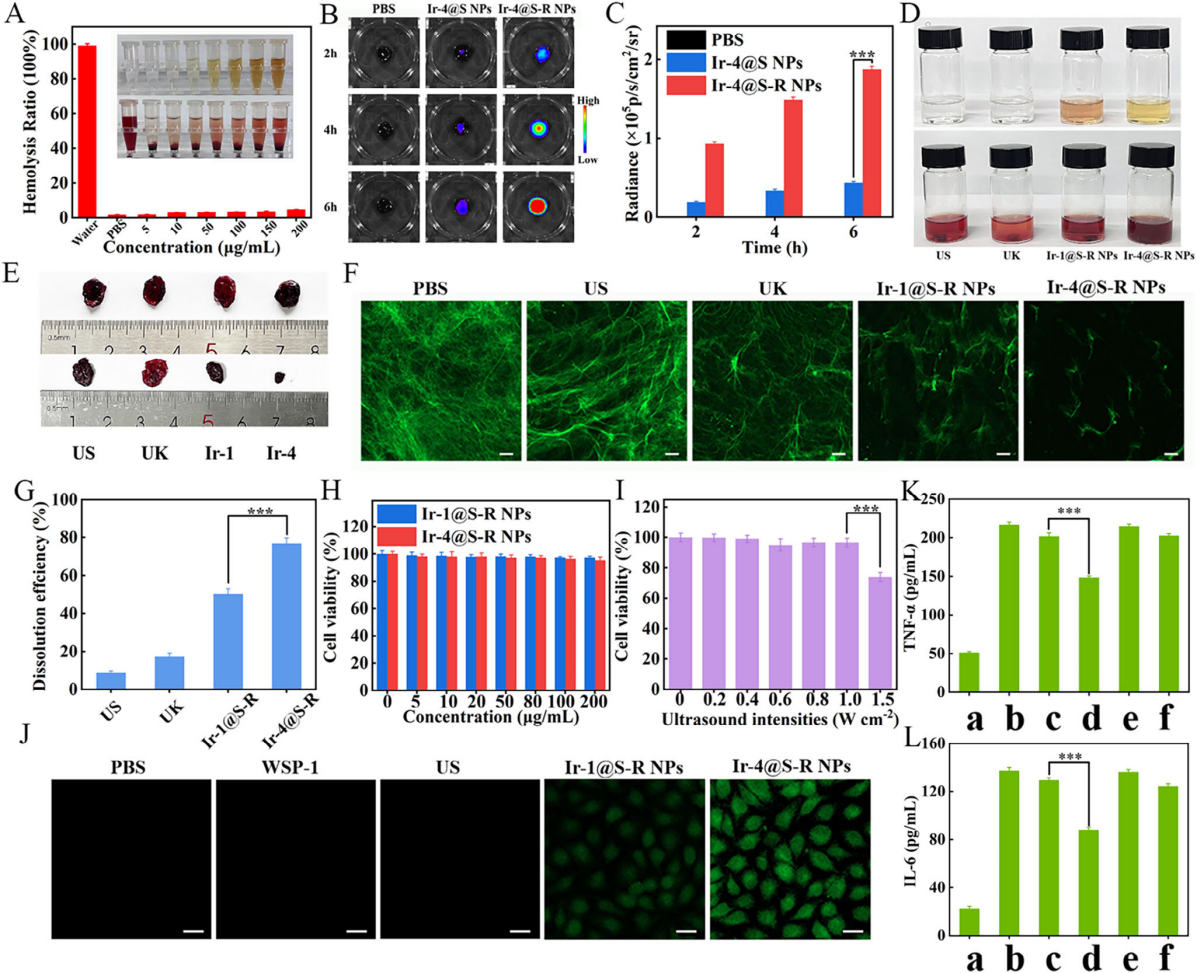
A) The hemolysis ratio of red blood cells treated with water, PBS and different concentrations of Ir‐4@S‐R NPs in water. Inset shows the red blood cells solution upon different treatments (from left to right: water, PBS, 5, 10, 50, 100, 150, and 200 µg mL^‒1^ of Ir‐4@S‐R NPs). Data are presented as mean ± SD (n = 3 independent experiments); B) The chemiluminescence images of artificial blood clots after incubation with PBS, Ir‐4@S NPs, or Ir‐4@S‐R NPs; C) Quantitative analysis of the relative persistent intensity of the artificial blood clots in B). Data are presented as mean ± SD (n = 3 independent experiments). p* < 0.05, *p* < 0.01, and p* < 0.001 versus control group; D)‐E) Photographs of the blood clot solution after different treatments (from left to right: ultrasound, free UK, Ir‐1@S‐R NPs + ultrasound, Ir‐4@S‐R NPs + ultrasound). The residual blood clots were taken out and shown; F) Fluorescence images of fibrin clots with different treatments. Scale bar: 100 µm; G) thrombolytic rates were quantitatively analyzed by measuring the percentage of thrombus mass loss after different treatments in E). Data are presented as mean ± SD (n = 3 independent experiments). p* < 0.05, *p* < 0.01, and p* < 0.001 versus control group; H) The cytotoxicity of different concentrations of Ir‐4@S‐R NPs in HUVECs. Data are presented as mean ± SD (n = 3 independent experiments). p* < 0.05, *p* < 0.01, and p* < 0.001 versus control group; I) The cytotoxicity of 10 min of ultrasound at different intensities in HUVECs. Data are presented as mean ± SD (n = 3 independent experiments). p* < 0.05, *p* < 0.01, and p* < 0.001 versus control group; J) Intracellular fluorescence imaging of H_2_S release in different treatment groups (PBS + WSP‐1, WSP‐1, ultrasound + WSP‐1, Ir‐1@S‐R NPs + ultrasound + WSP‐1, Ir‐4@S‐R NPs + ultrasound + WSP‐1), Scale bar: 50 µm; K) TNF‐α and L) IL‐6 in HUVECs by enzyme‐linked immunosorbent assay (a: PBS, b: LPS, c: LPS + Ir‐1@S‐R NPs, d: LPS + Ir‐4@S‐R NPs, e: LPS + PAG + Ir‐1@S‐R NPs, f: LPS + PAG + Ir‐4@S‐R NPs). Data are represented as mean ± SD (n = 3). The data were analyzed by unpaired 2‐tailed Student's *t*‐test using GraphPad Prism 7 (C, G, I, K and L). p* < 0.05, *p* < 0.01, p* < 0.001.
